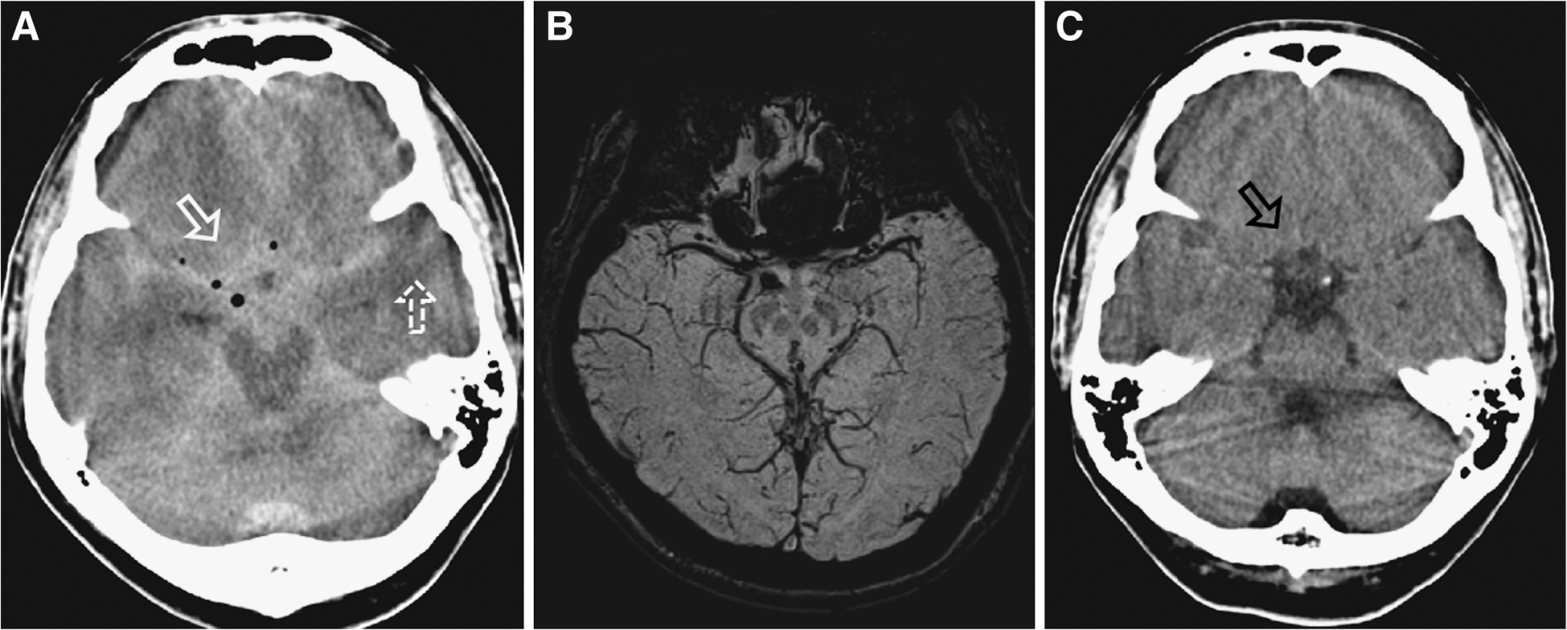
Cranial images. (A) The contrast filling the subarachnoid space with intracranial air (white arrow) and diffuse parenchymal swelling (white dotted arrow), mimicking a subarachnoid hemorrhage. (B) Subtractional magnetic resonance imaging showed no vascular malformation or intracranial hemorrhage. (C) The washed-out subarachnoid space after conservative management for one day (black arrow).
